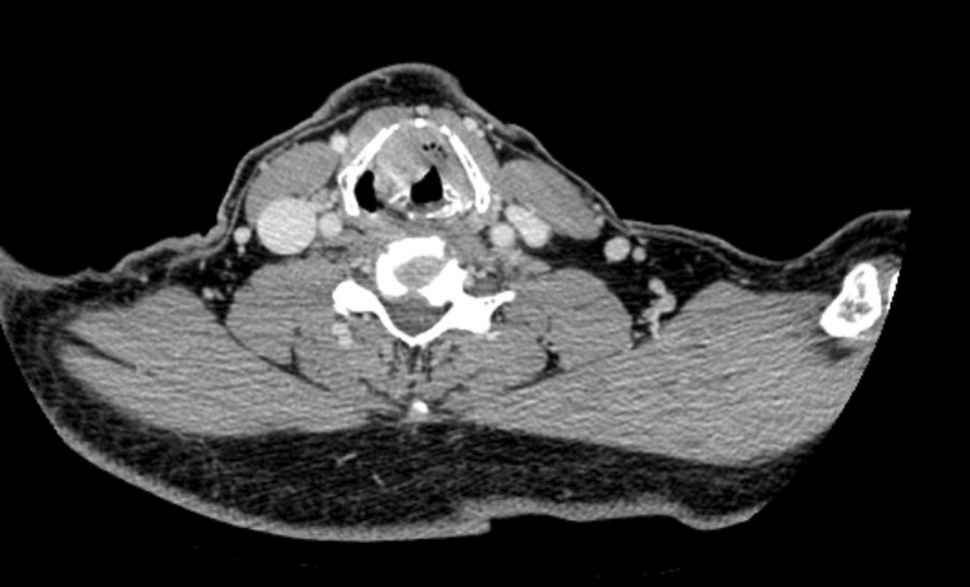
Preoperative enhanced CT images of one of the patients: the laryngeal cancer with slight enhancement has already penetrated and destructed the thyroid cartilage
